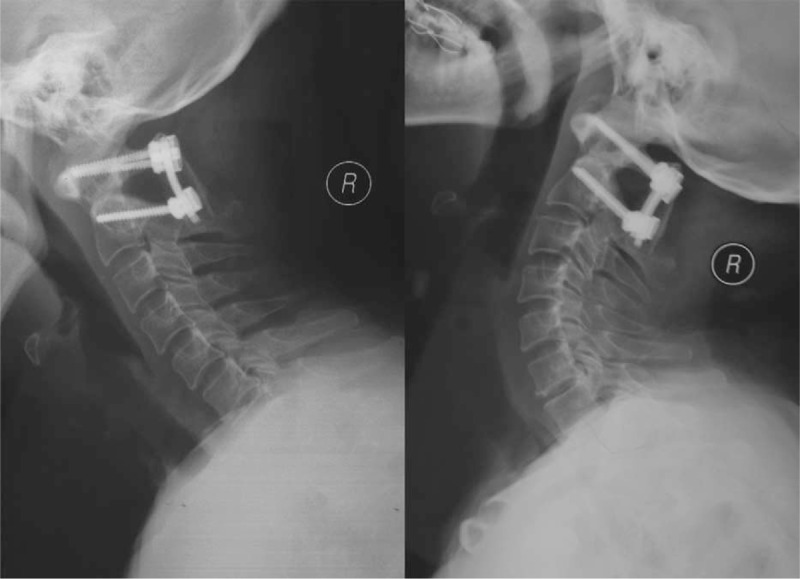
Dynamic lateral X-ray demonstrated stability of the atlantoaxial complex with solid autograft fusion at 6 months postoperation follow-up.
